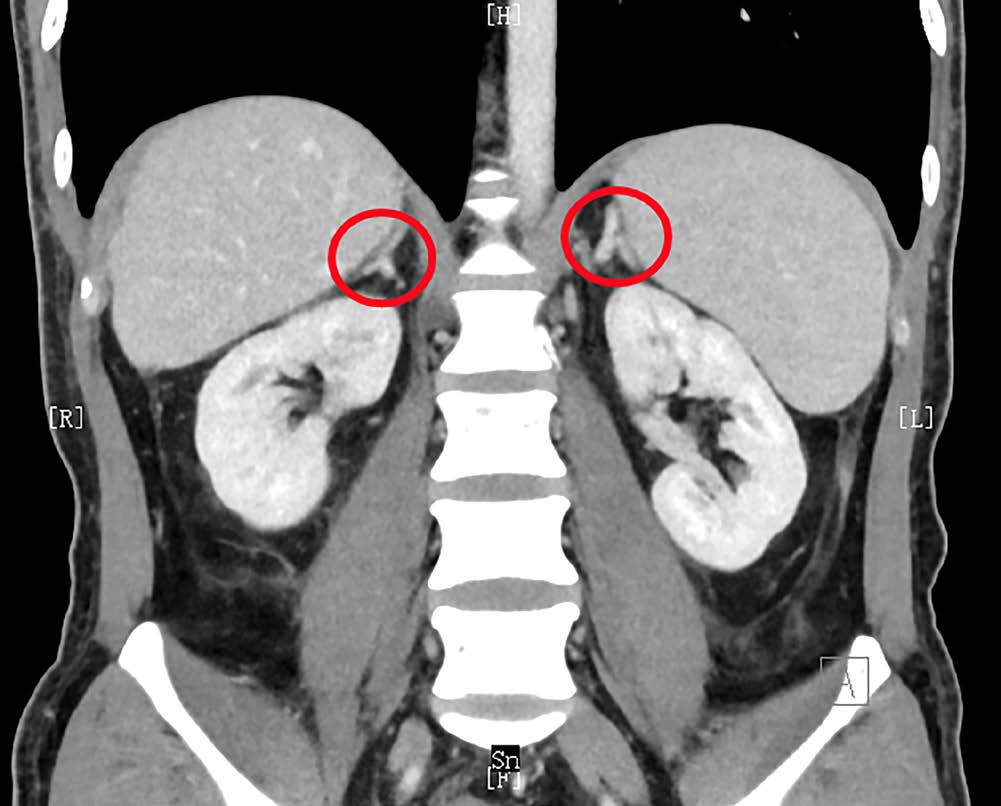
CT reexamination after 14 weeks of treatment showed no progress.
